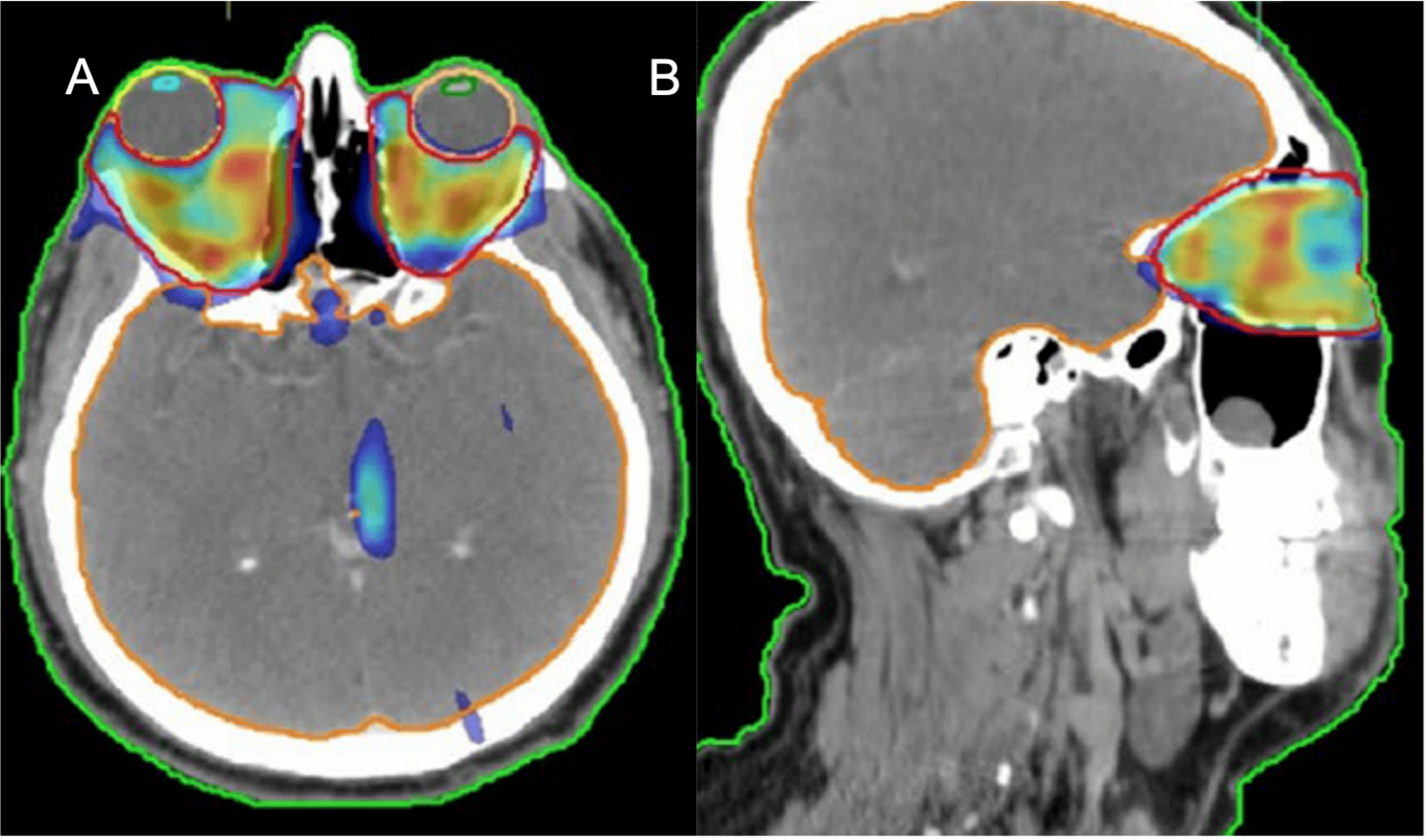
A 51-year-old woman diagnosed with Graves' orbitopathy underwent orbital radiotherapy using the IMRT technique, with a total dose of 20 Gy in 10 fractions a: Axial CT section showing the orbital structures and treatment planning. b: Sagittal section showing the dose distribution and target coverage. IMRT: intensity-modulated radiotherapy, CT: computed tomography
